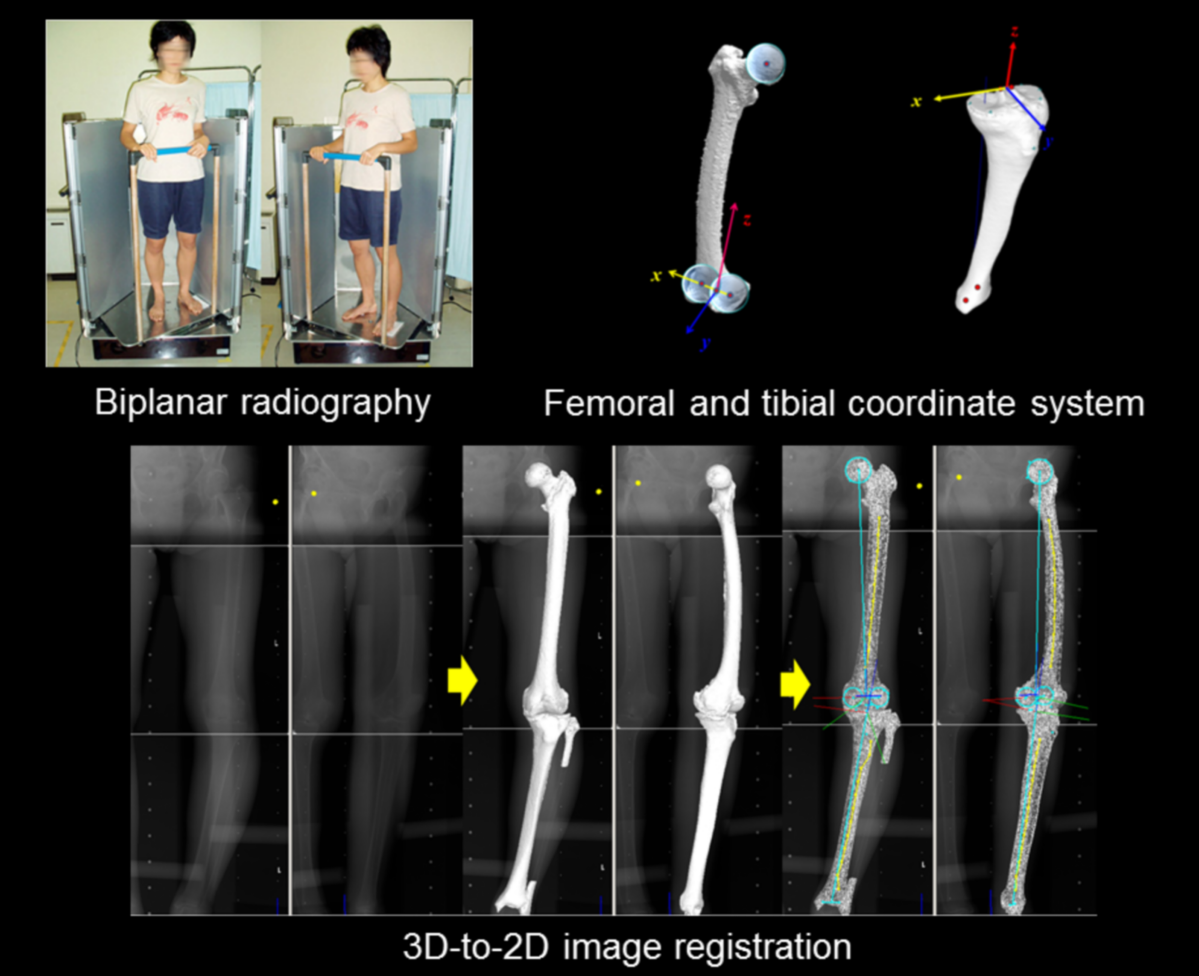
The biplanar radiography images of all the lower leg extremities under WB conditions. The 3D-to-2D image registration technique enabled the projection of the 3D digital bone models from the CT data, incorporating the anatomical coordinate system and the information of the morphology and alignment, onto biplanar radiography images.
